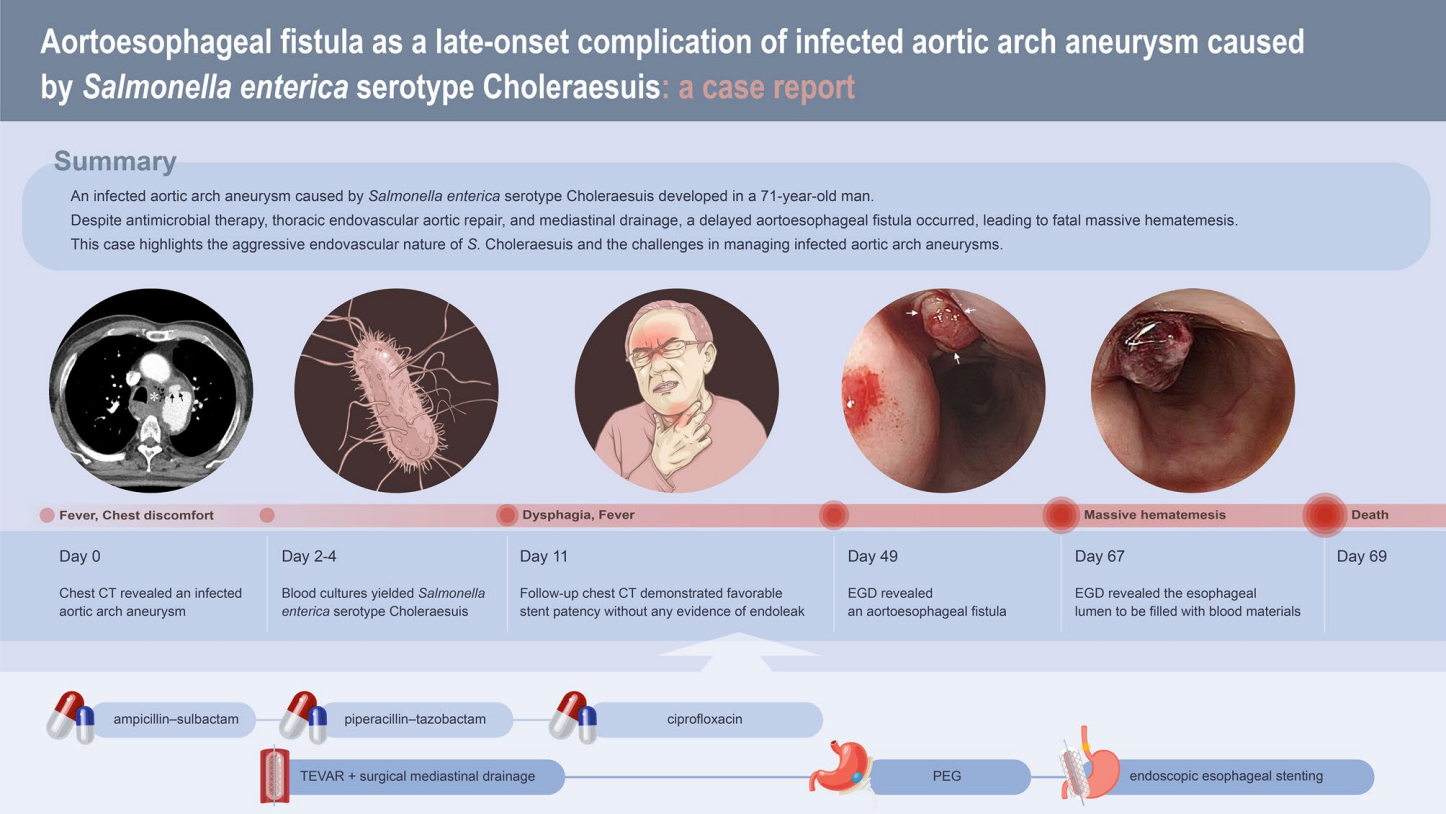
Graphical summary of the clinical course. CT = computed tomography, EGD = esophagogastroduodenoscopy, TEVAR = thoracic endovascular aortic repair
